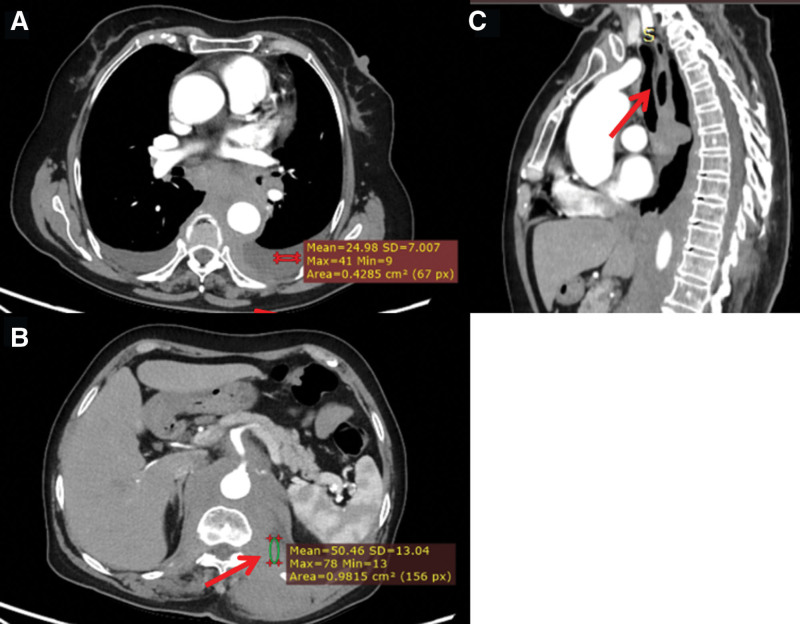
Contrast-enhanced CT images de1monstrating extensive infiltrative lesions (A) middle and posterior mediastinum; (B) celiac trunk and retroperitoneum space; (C) esophagus. CT = computed tomography.
